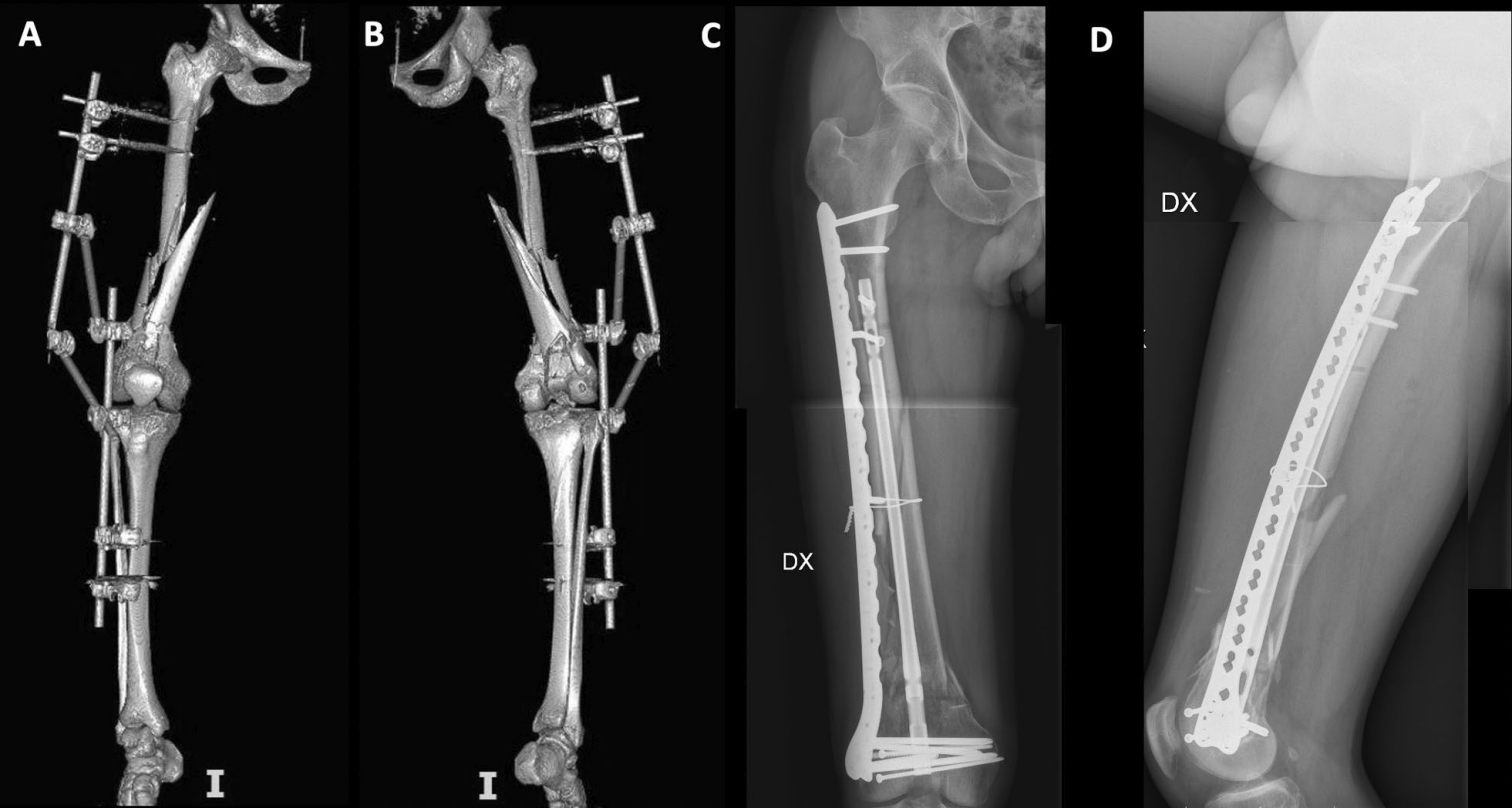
Preoperative three-dimensional (3D) CT reconstructions in anterior view (A) and posterior view (B), and postoperative radiographs in anteroposterior view (C) and lateral view (D) of a patient treated with a nail–plate construct (NPC)
